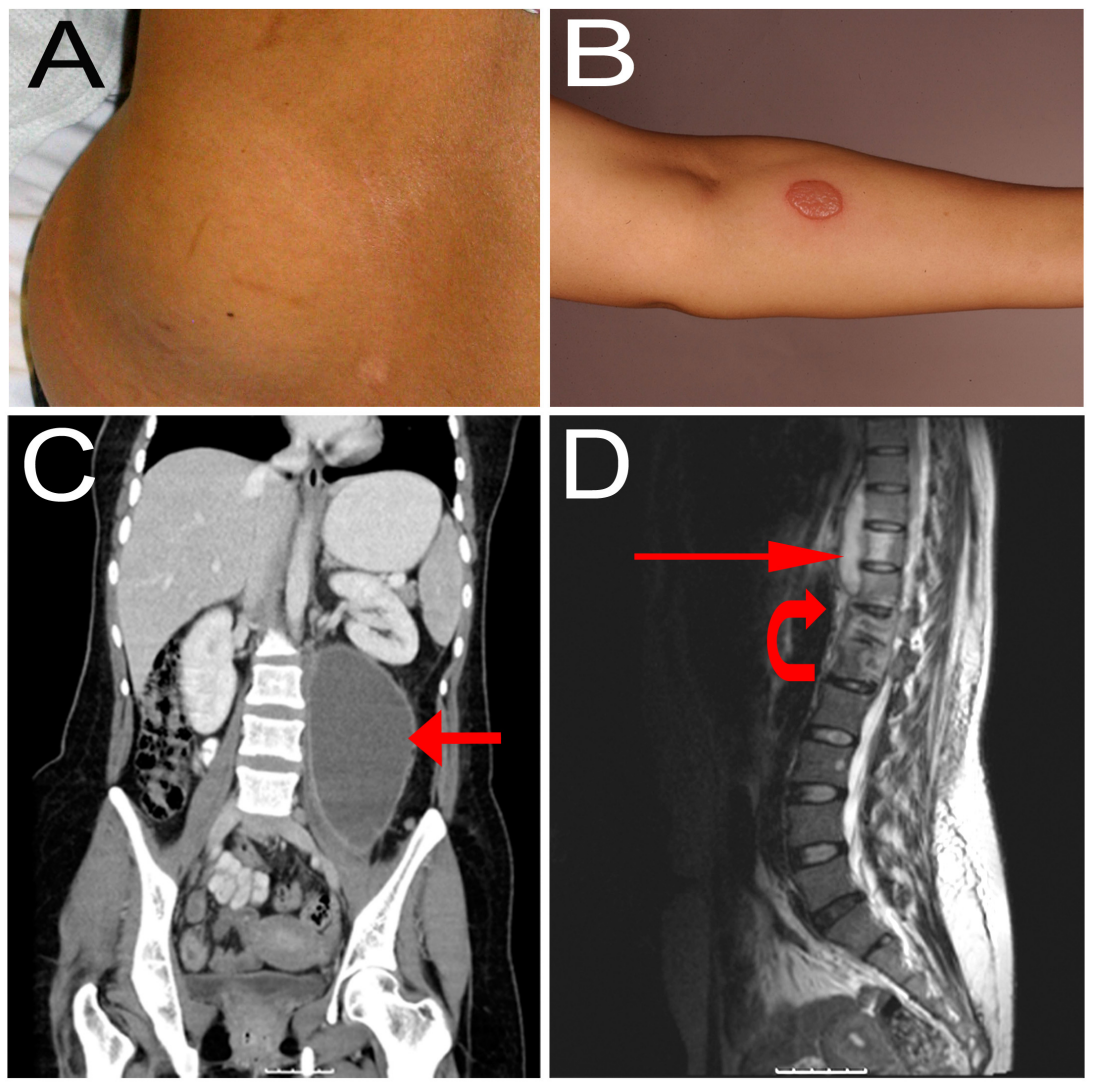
A) Subcutaneous mass in the left loin. B) Result of Mantoux test eliciting a strongly positive skin reaction. C) Coronal computed tomography scan with contrast showing large left psoas abscess (red arrow). D) T2-weighted sagittal magnetic resonance imaging scan showing paravertebral psoas collection (straight red arrow) and extensive involvement of T12 and L1 vertebral bodies with signal change and loss of intervertebral disc space at this level (within bounds of curved red arrow).
